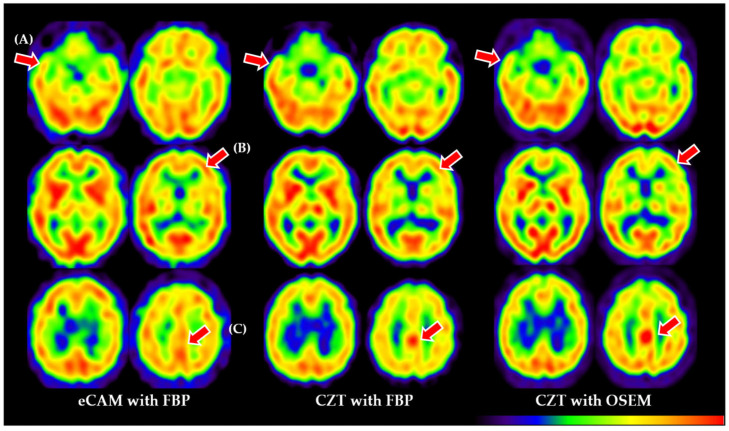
Representative brain scans showing key differences between eCAM with FBP (left), CZT with FBP (center), and CZT with OSEM (right). Red arrows indicate (A) improved temporal lobe definition in CZT images; (B) enhanced gray/white matter contrast, with sharper boundaries visible in CZT images; (C) different visualization of the posterior cingulate gyrus, a critical region for Alzheimer’s disease diagnosis. Note the progressively improved image clarity from left to right, with CZT OSEM reconstruction providing the highest definition of anatomical structures. eCAM = Brand name for single-photon emission computed tomography camera; FBP = Filtered back projection; CZT = Cadmium–zinc–telluride; OSEM = Ordered-subset expectation maximization.
